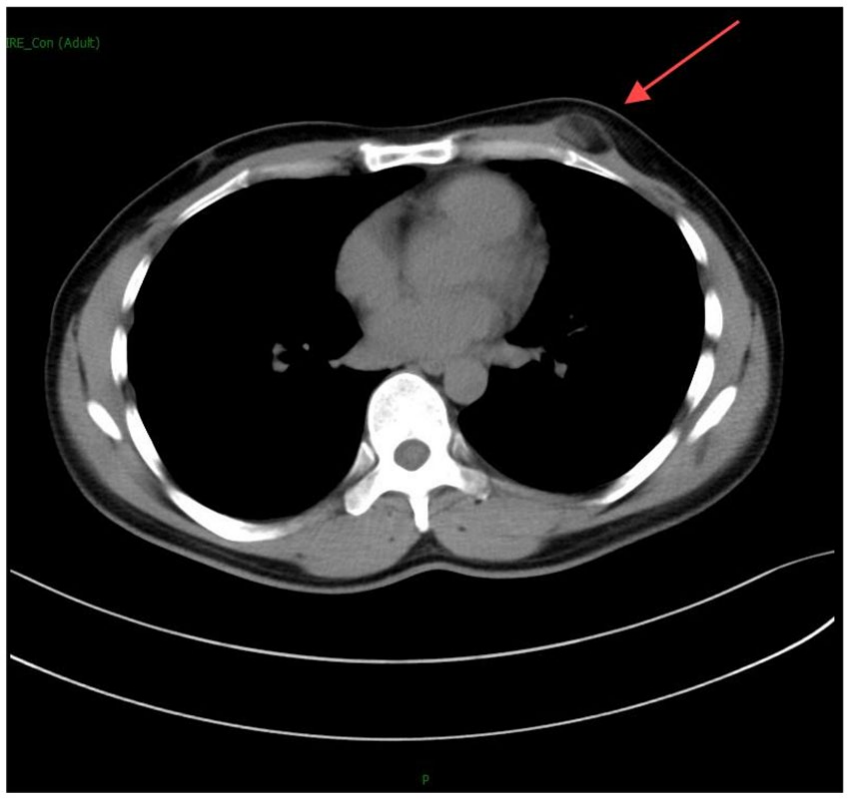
Chest CT scan showing a subcutaneous nodule measuring approximately 15 mm × 25 mm with fat density in the left chest wall. The nodule’s margins appear slightly blurred.
